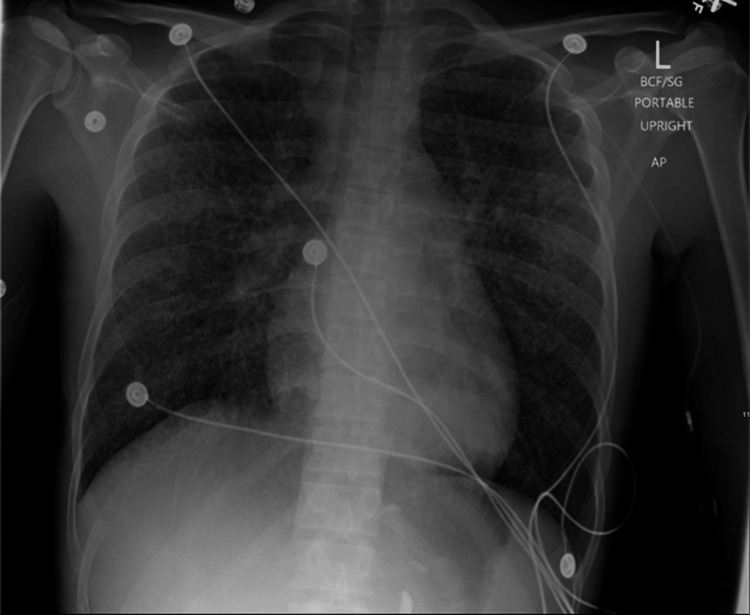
Chest X-ray upon readmission showing innumerable miliary pulmonary nodules and diffuse ground glass infiltrates, appearing worse compared to the chest X-ray upon initial admission.
